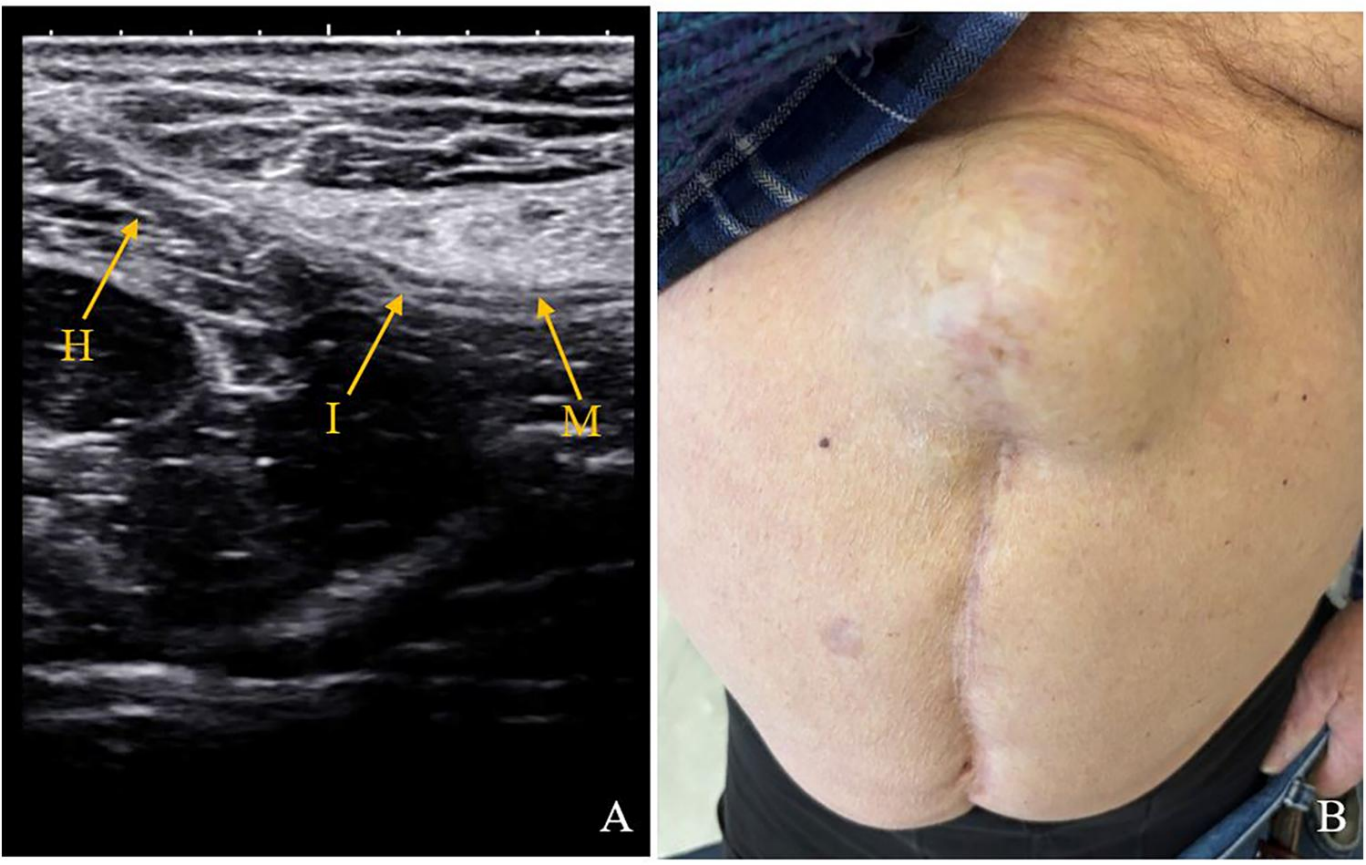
Ultrasound and clinical images of an 83-year-old patient who underwent re-laparotomy on day 13th postoperatively for hemo-peritoneum. (A) Ultrasound image at 23 months post operatively with incisional hernia. (B) Clinical image of a 8 cm incisional hernia. M, mesh; I, mesh interruption; H, hernia.
